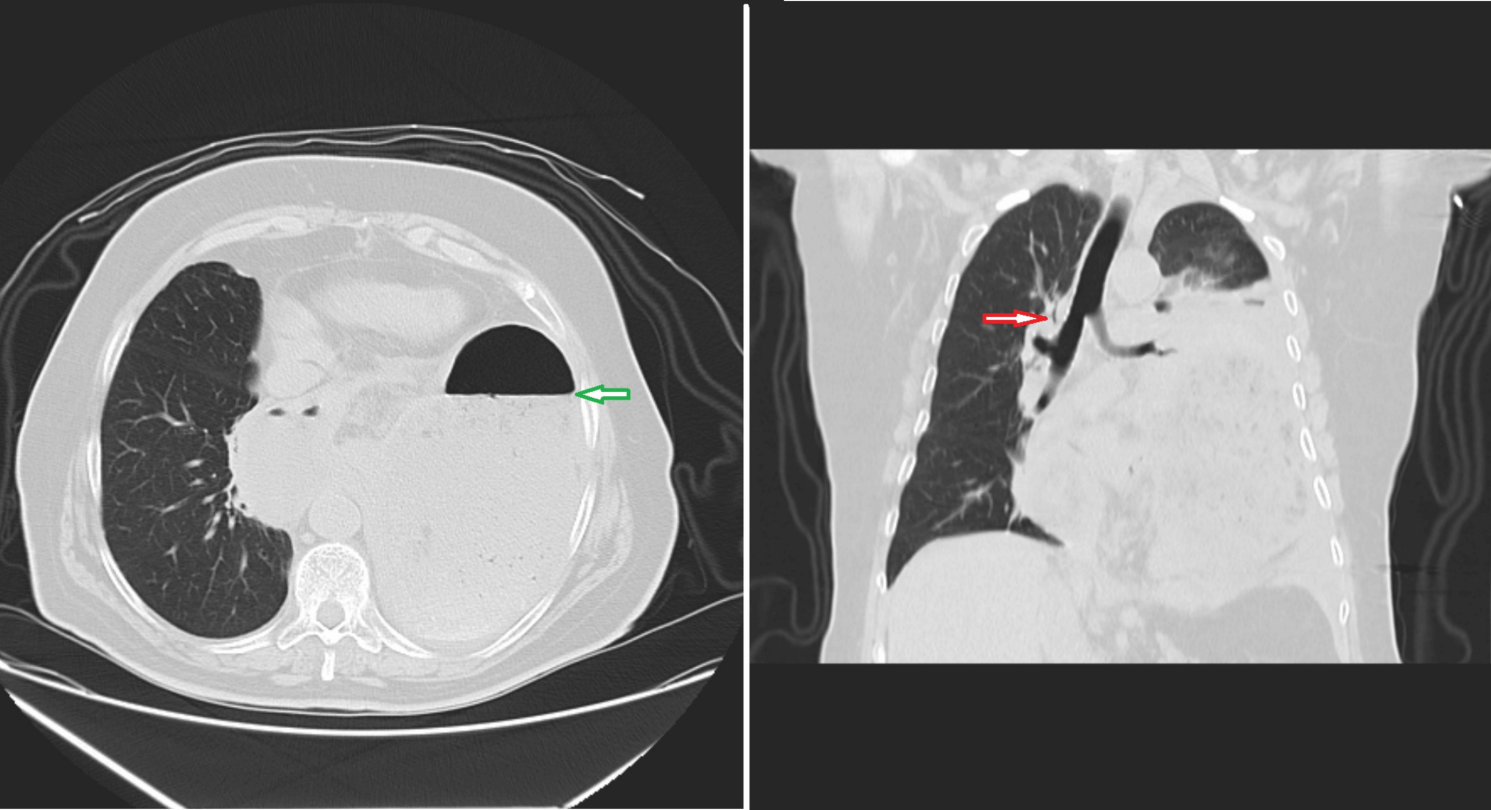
Thoracic CT scan showing an air-fluid level (green arrow) and right mediastinal shift (red arrow)
